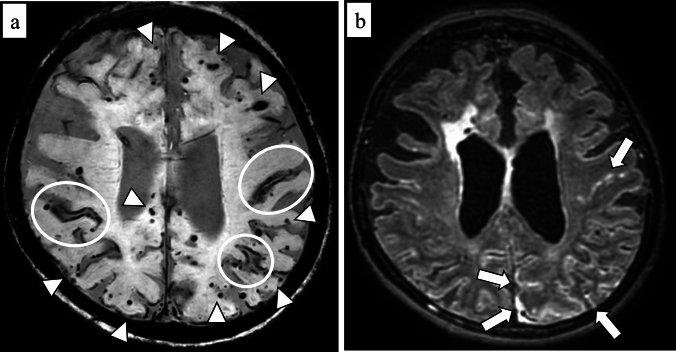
A 47-year-old female with iatrogenic CAA. MMSE score is 14. She had a history of cerebellar tumor resection when she was 5 years old. SWI (a) shows lobar CMBs (arrowheads) and cSS (circles). Contrast-enhanced 3D FLAIR (b) shows leptomeningeal enhancement in the left parieto-occipital lobe (arrows)
